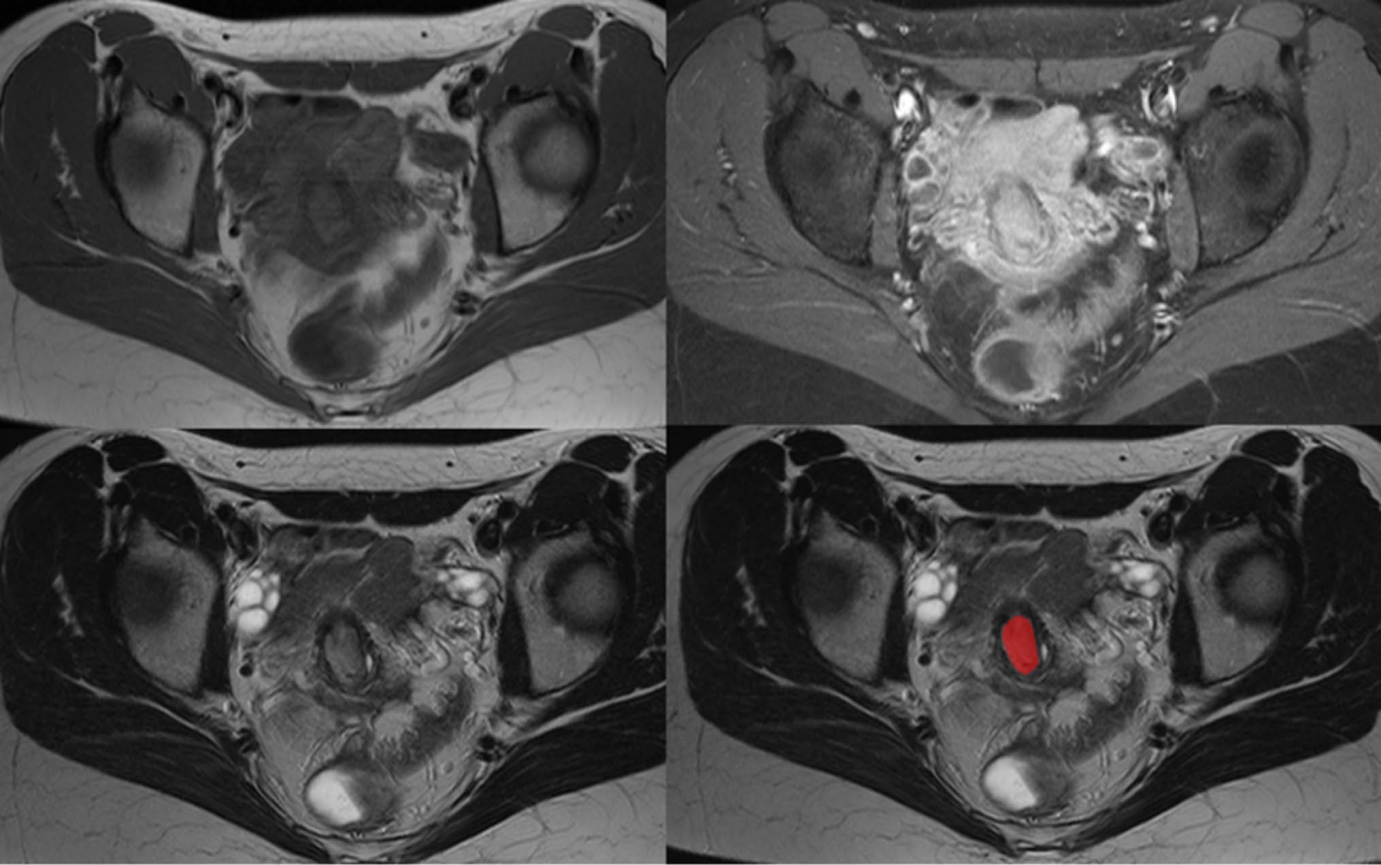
Representative patient of the present cohort, FIGO IB1, T1b1 N0 M0, G1. A. T1-weighted axial image showing the small inhomogeneous tumor with intraluminal extension. B. T1-weighted axial image contrast media application with fat-saturation. The tumor shows a remarkable contrast media uptake C. T2-weighted axial sequence. D. Drawn region of interest within the boundaries of the tumor displayed in red
